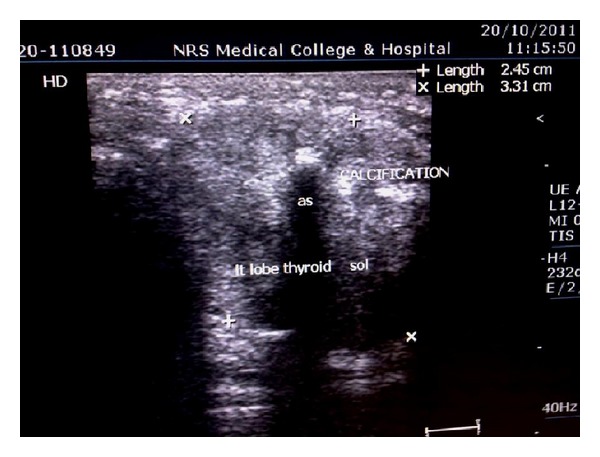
Ultrasonogram of thyroid showing calcifications with hypoechoic lesion (label).
